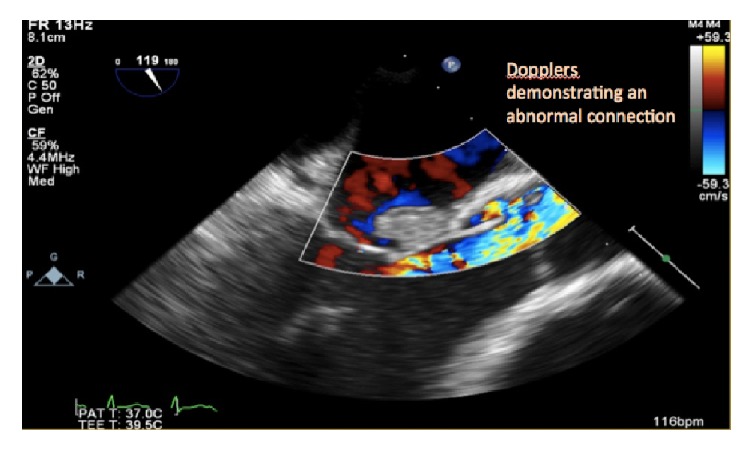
Doppler confirming an abnormal flow tract from the aorta.
